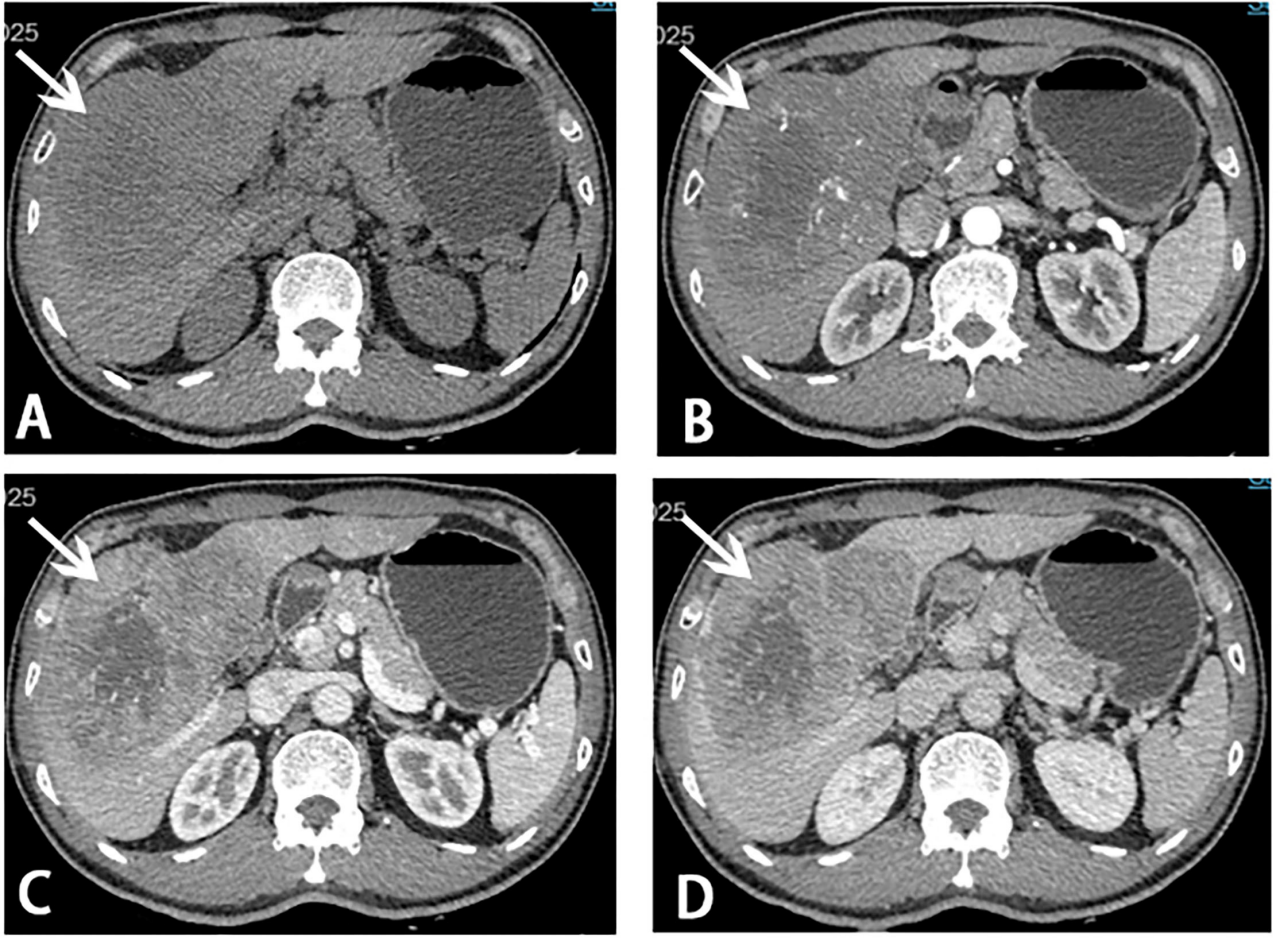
Computed tomography (CT) scans before stage I ALPPS. A giant mass (white arrow) measuring 13.3 × 8.5 × 13.0 cm in liver segments 4, 5, 6, 7, and 8 was showed on CT scans. (A) Plain scan; (B) arterial phase; (C) portal venous phase; (D) venous phase.
